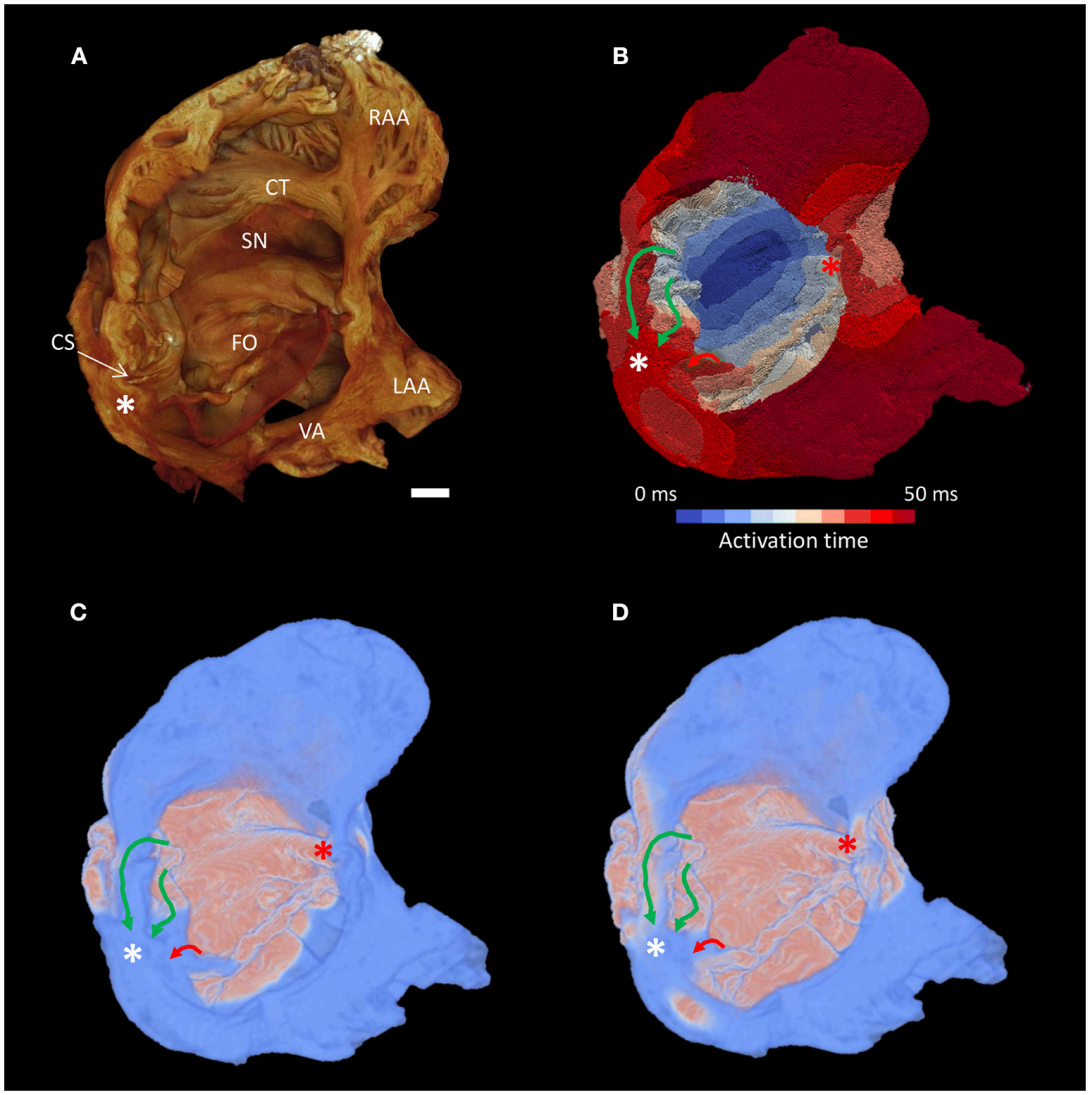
Preferential inter-nodal conduction via the “slow” pathway in the atria of a heart with AVSD. (A) Volume rendering of the atrial cavity viewed from the inferior-lateral position. (B) Corresponding isochrone electrical activation map, the normal location and direction of the “slow” pathway (green), and the septal aspect of the elongated “fast” pathway (red) are indicated by solid arrows. (C,D) Snapshots taken from the Supplementary Video 2 showing excitation of the distal aspect of the region normally associated with the “slow” pathway precedes that of the “fast” pathway, pink indicates activated myocardium, light blue indicates dormant myocardium. See methods for modeling parameters. White*, location of compact atrioventricular node in AVSD heart; Red*, approximate location of compact atrioventricular node in normal heart; CS, coronary sinus; CT, terminal crest; FO, fossa ovale; LAA, left atrial appendage; RAA, right atrial appendage; SN, sinus node; VA, valve annulus. Scale bar represents 3 mm.
